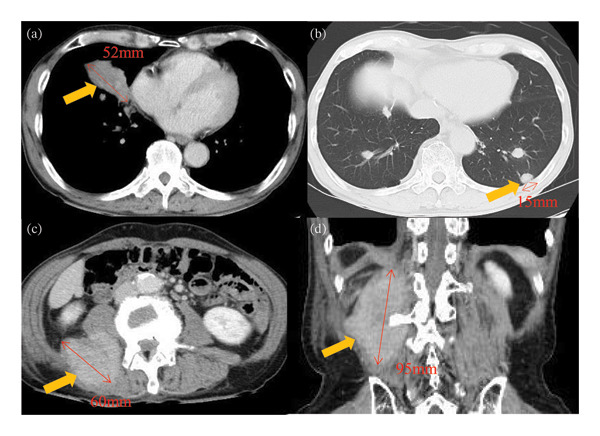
CT images obtained upon admission. (a) CE chest CT reveals a mass in the right middle lobe with a tumor major axis of 52 mm (arrow). (b) Chest CT reveals multiple nodules in both lung fields. The major axis of the tumor in contact with the pleura measured 15 mm (arrow). (c) CE axial abdominal CT reveals a mass in the right psoas muscle with the tumor’s major axis measuring 60 mm (arrow). (d) CE coronal abdominal CT reveals a mass in the right psoas with the tumor’s major axis measuring 95 mm (arrow). CE: contrast‐enhanced; CT: computed tomography.
